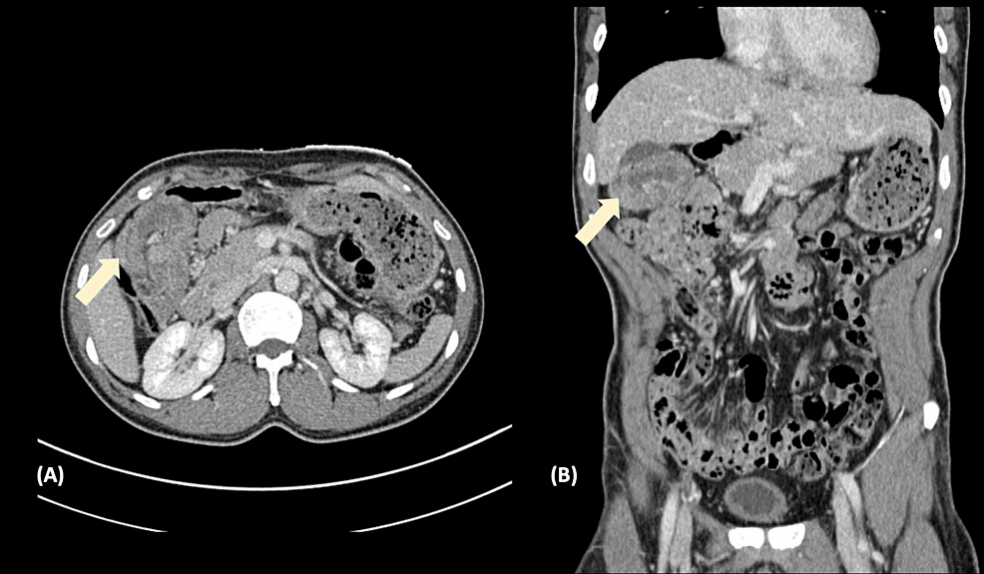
Computed tomography showing intussusception at the hepatic flexure, with the intussusceptum containing a low-attenuation mass (arrow) in the (A) axial view and (B) coronal plane.
